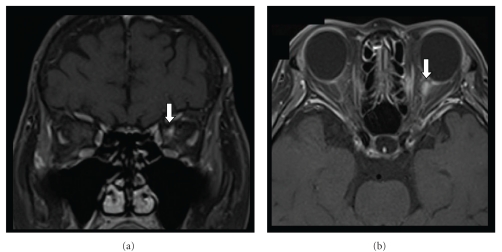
Brain magnetic resonance images with T1-weighted gadolinium enhancement performed 1 week after the onset of left visual loss. A high-intensity lesion on the left optic nerve is seen (white arrows).
